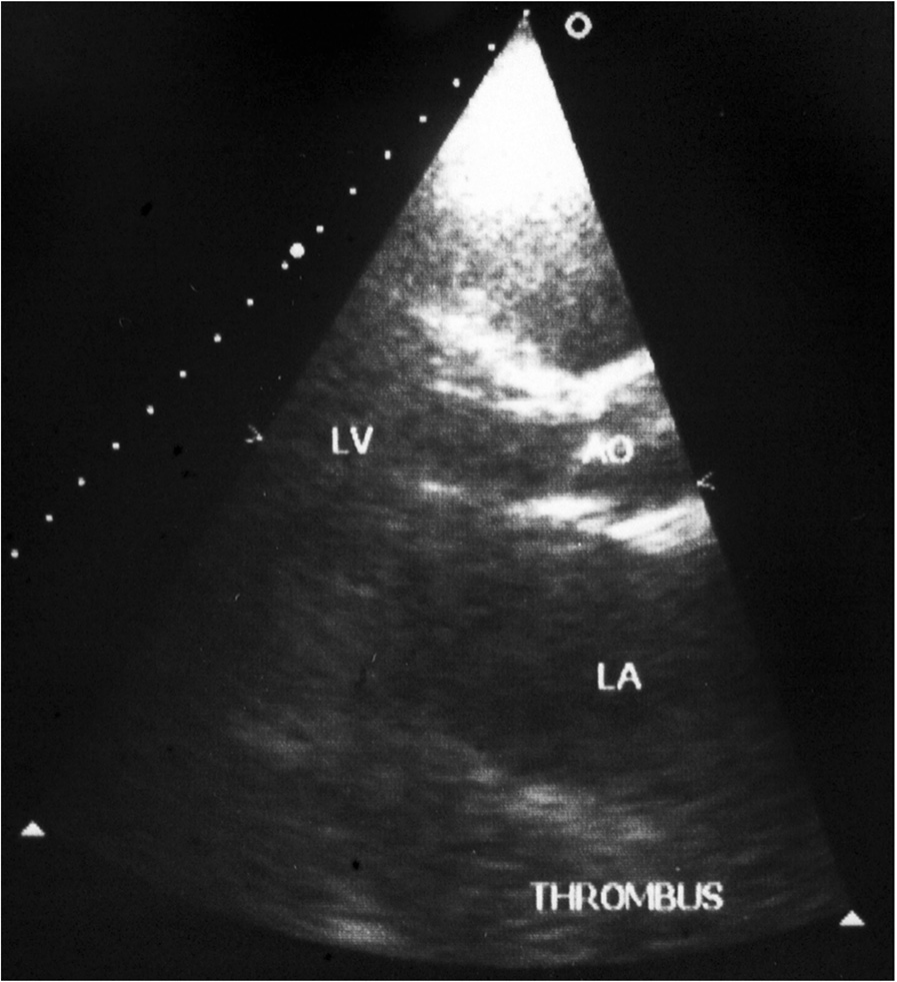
Preoperative echocardiography showing a huge left atrium occupied by a thrombus.
